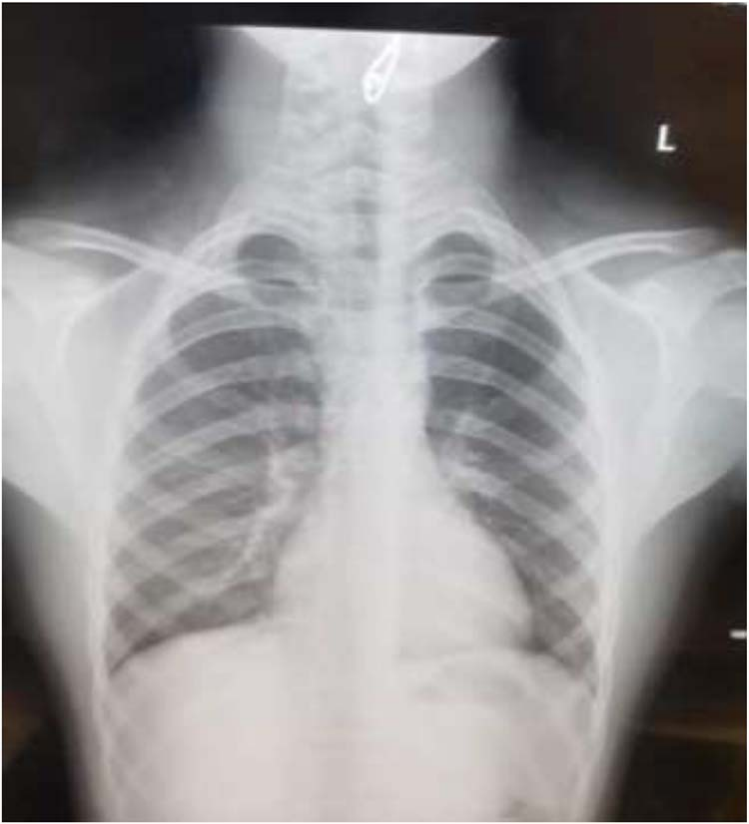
AP chest radiograph showing the sharp-edged radio-opaque object.
